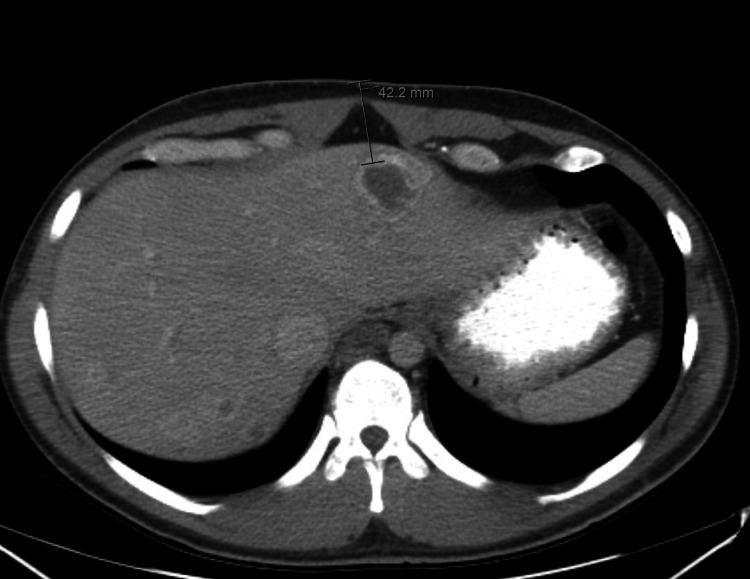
CT of the abdomen showing hepatic metastatic lesions at the time of presentation and prior to chemotherapy initiation
